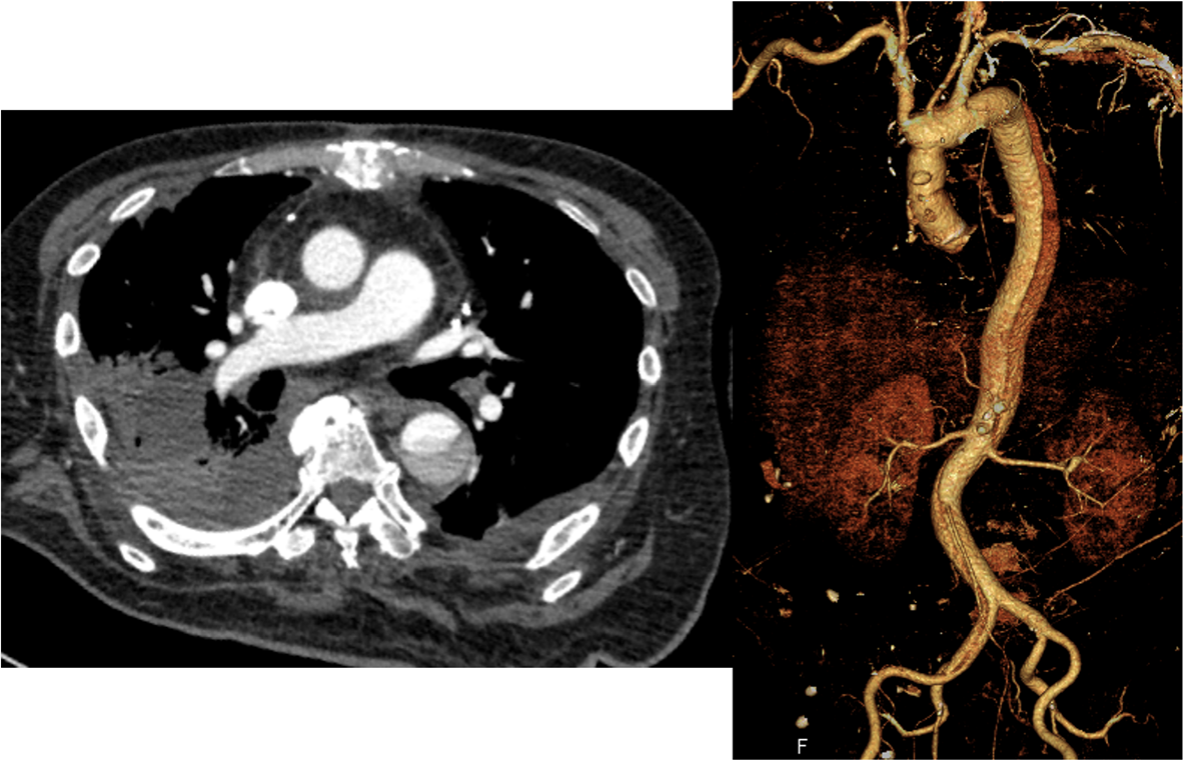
Postoperative enhanced-computed tomography (CT) images
